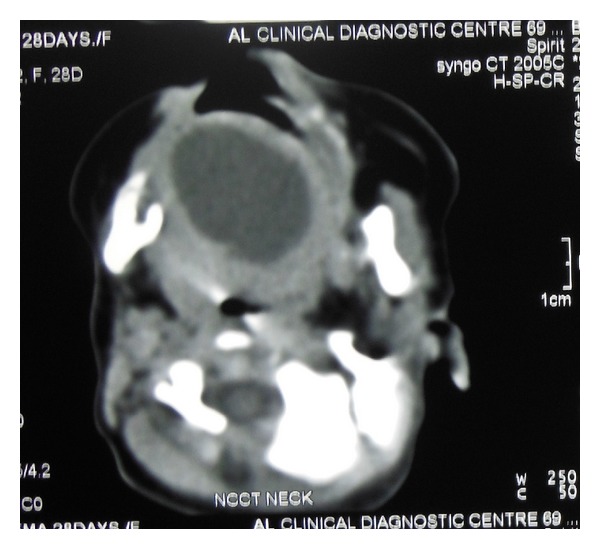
CT scan showing the cystic lesion in the axial cut.
